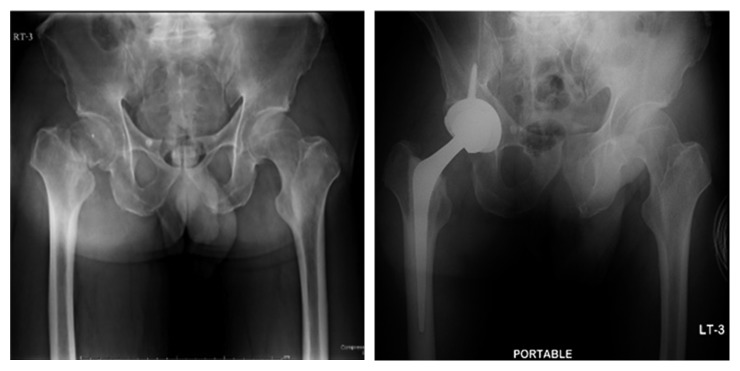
Radiograph of the hip before surgery (left) and after surgery (right).
